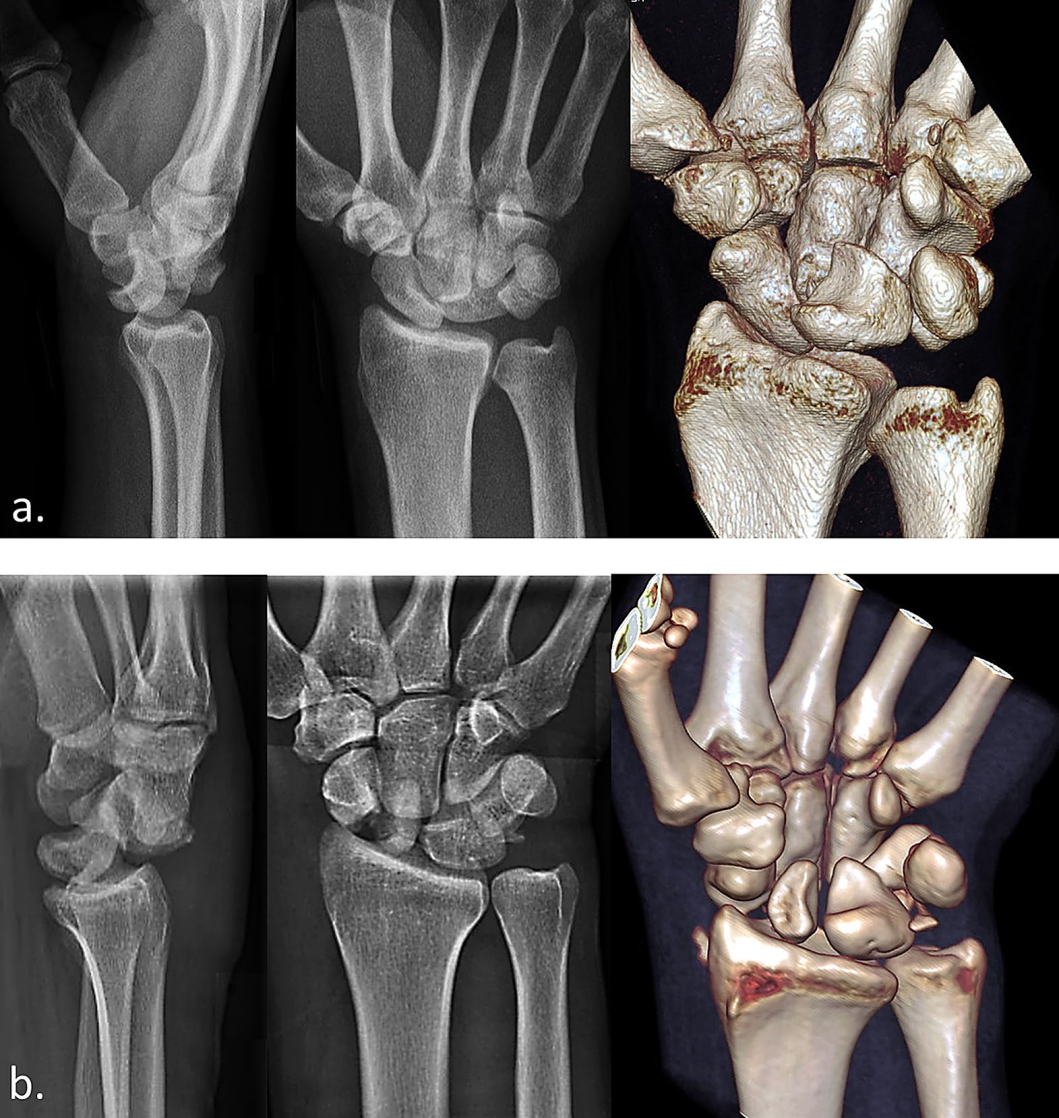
X-ray series in lateral and PA projections, along with corresponding CT scans with three-dimensional reconstructions of a ligamentous-only perilunate dislocation (a) and a trans-scaphoid perilunate fracture-dislocation (b)
